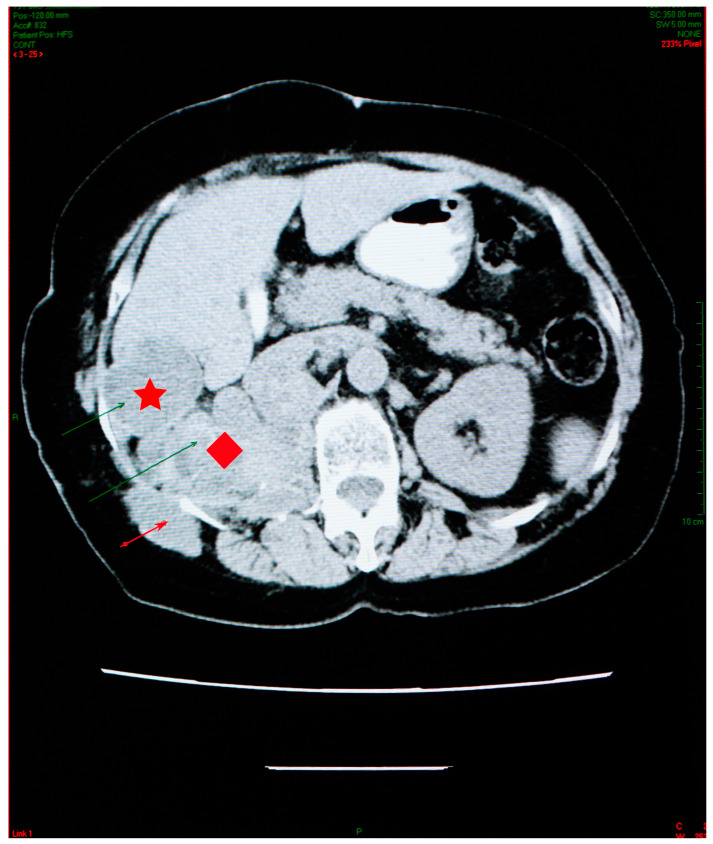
Abdominal CT shows reduction in the aspect and dimensions of the cysts—right kidney space (red diamond), the sixth right liver segment (star) and in the right iliac fossa and right paravertebral area (arrow)—associated with favorable evolution under treatment.
